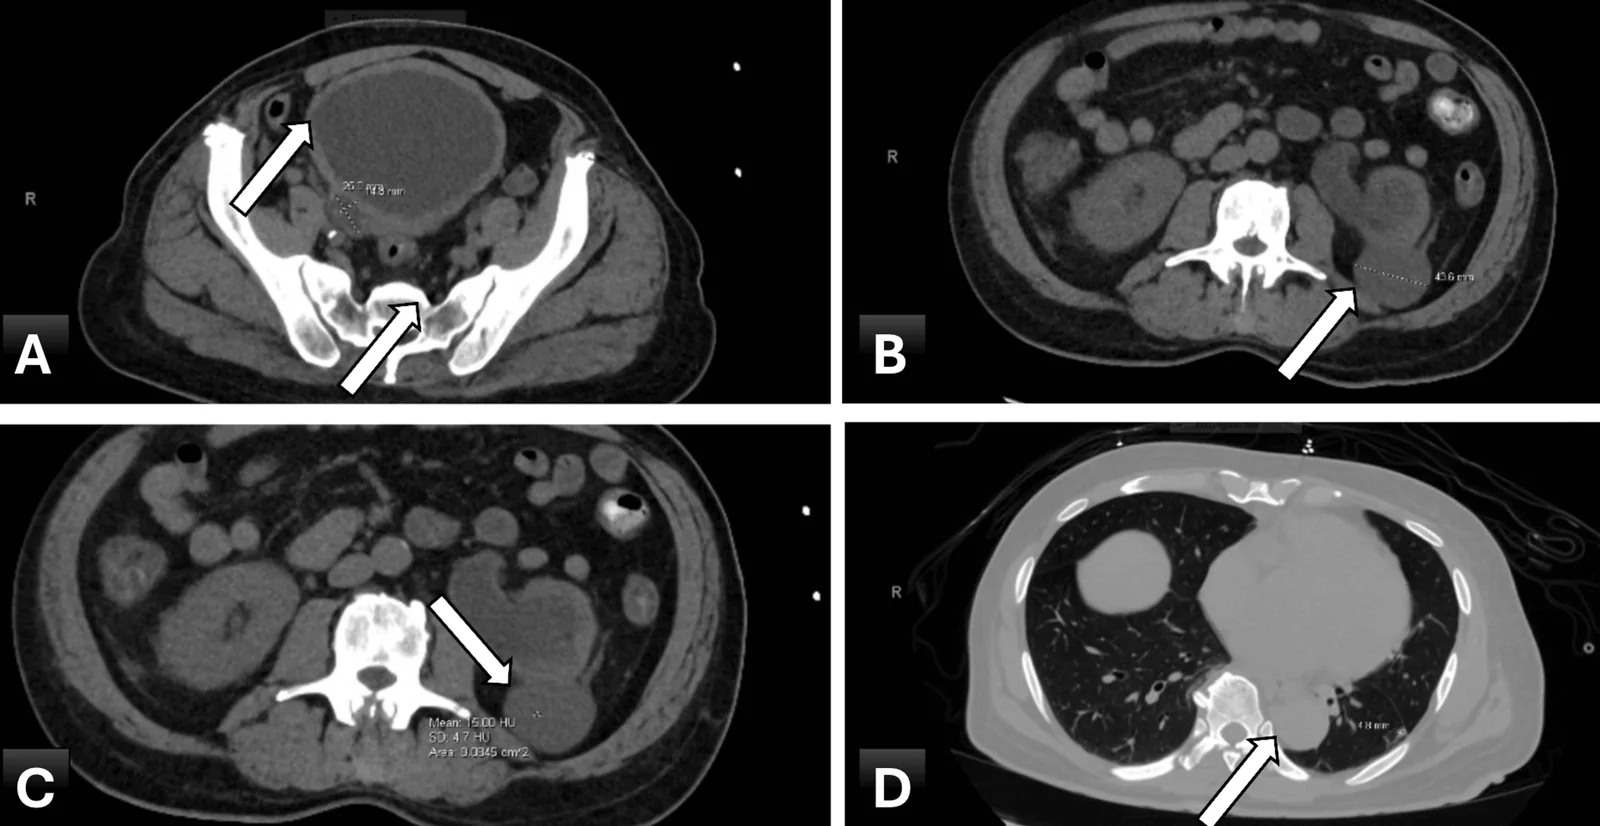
Computed tomography of the abdomen and pelvis demonstrating chronic obstructive uropathy and associated urinary tract abnormalities. (A) Axial CT image demonstrating marked bladder distention with diffuse circumferential bladder wall thickening and trabeculation (upper arrow) in the setting of chronic bladder outlet obstruction. The suprapubic catheter is also visible (lower arrow). (B) Axial CT image demonstrating a simple left lower pole renal cyst (arrow). (C) Axial CT image showing severe chronic left hydroureteronephrosis with marked renal cortical thinning (arrow), consistent with longstanding obstructive nephropathy. (D) Lung window image demonstrating a small left lower lobe perifissural pulmonary nodule (approximately 5 mm, arrow), considered an incidental finding.
